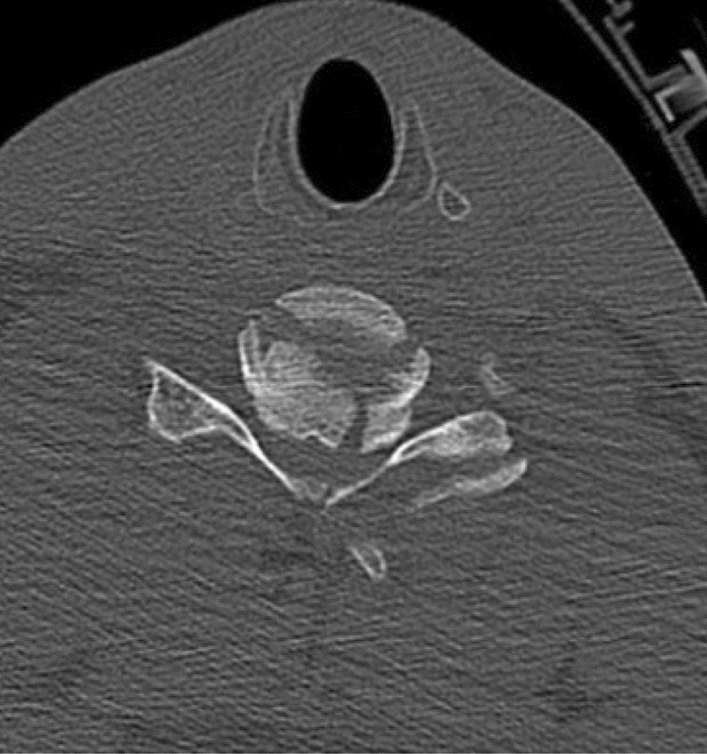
Burst fracture, CT scan axial view
